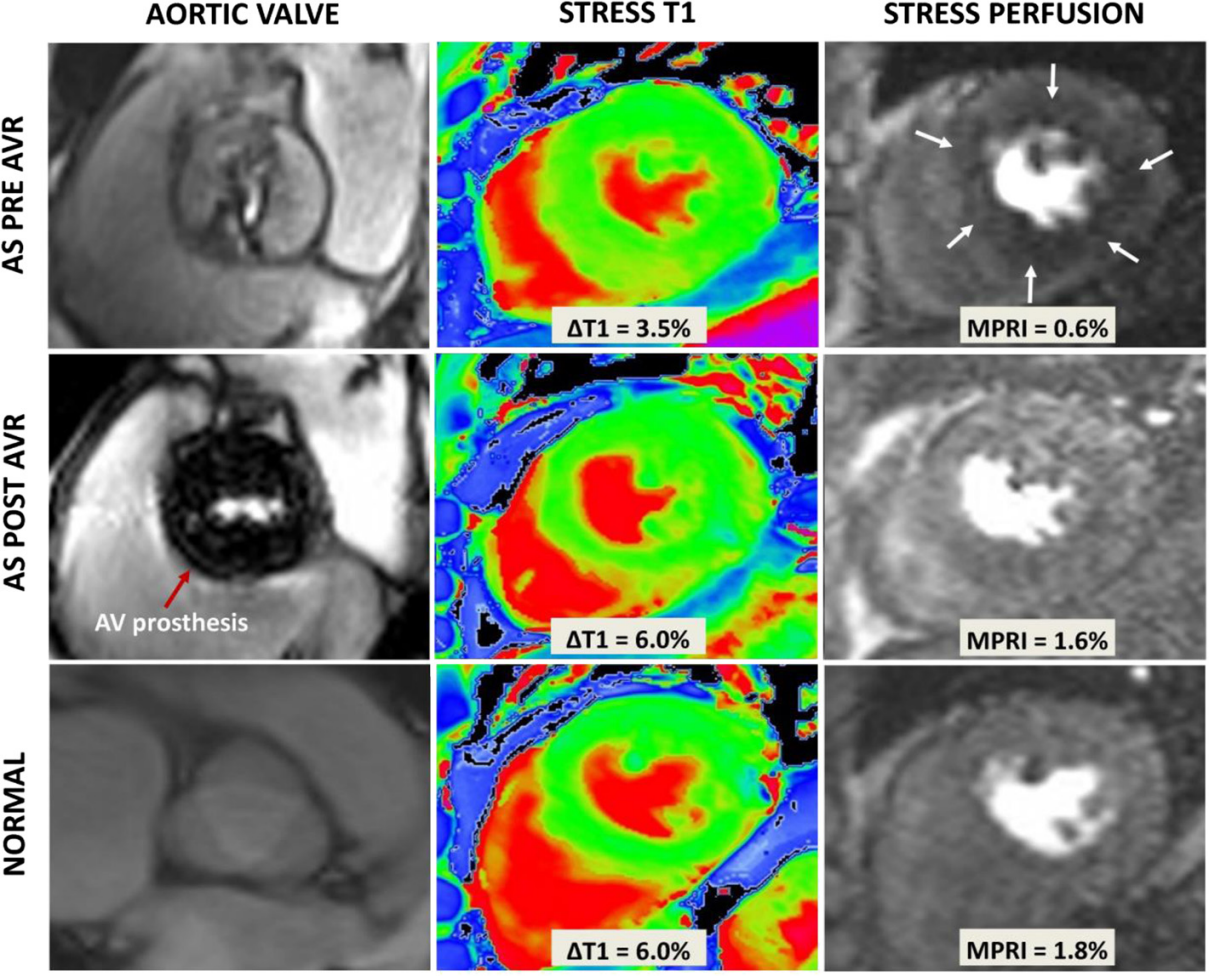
Examples of stress T1 imaging. Cardiovascular magnetic resonance images show examples of AS pre and post AVR, and normal (from top to bottom panels). From left to right, examples of AV cine, stress T1 (with ∆T1 values between rest and stress), and stress perfusion imaging (with MPRI values). White arrows show circumferential perfusion defect in AS pre AVR which is not seen in post AVR or normal. AS = aortic stenosis; AVR = aortic valve replacement; AV = aortic valve; ∆T1 = change in T1; MPRI = myocardial perfusion reserve index.
